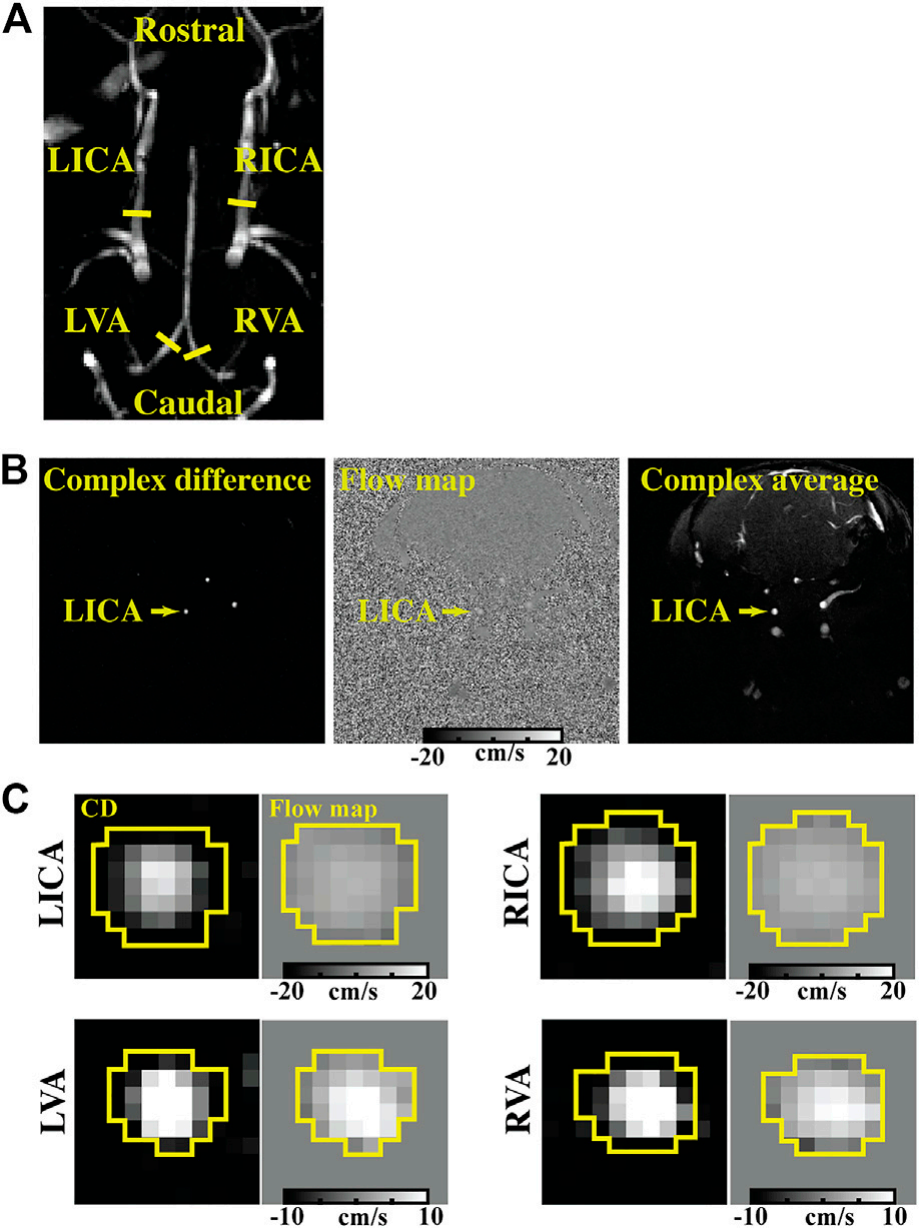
Representative dataset of PC MRI. (A) shows the TOF image displaying the slice locations of PC MRI (yellow solid lines perpendicular to the vessel trajectory) focusing on different arteries (LICA, RICA, LVA, and RVA). (B) shows the complex difference (CD), flow map, and complex average images of a PC scan focusing on the LICA. (C) presents the CD images and flow maps of four feeding arteries. ROIs for quantifying blood flow were delineated on the CD images (yellow boxes) and then applied to the flow maps.
